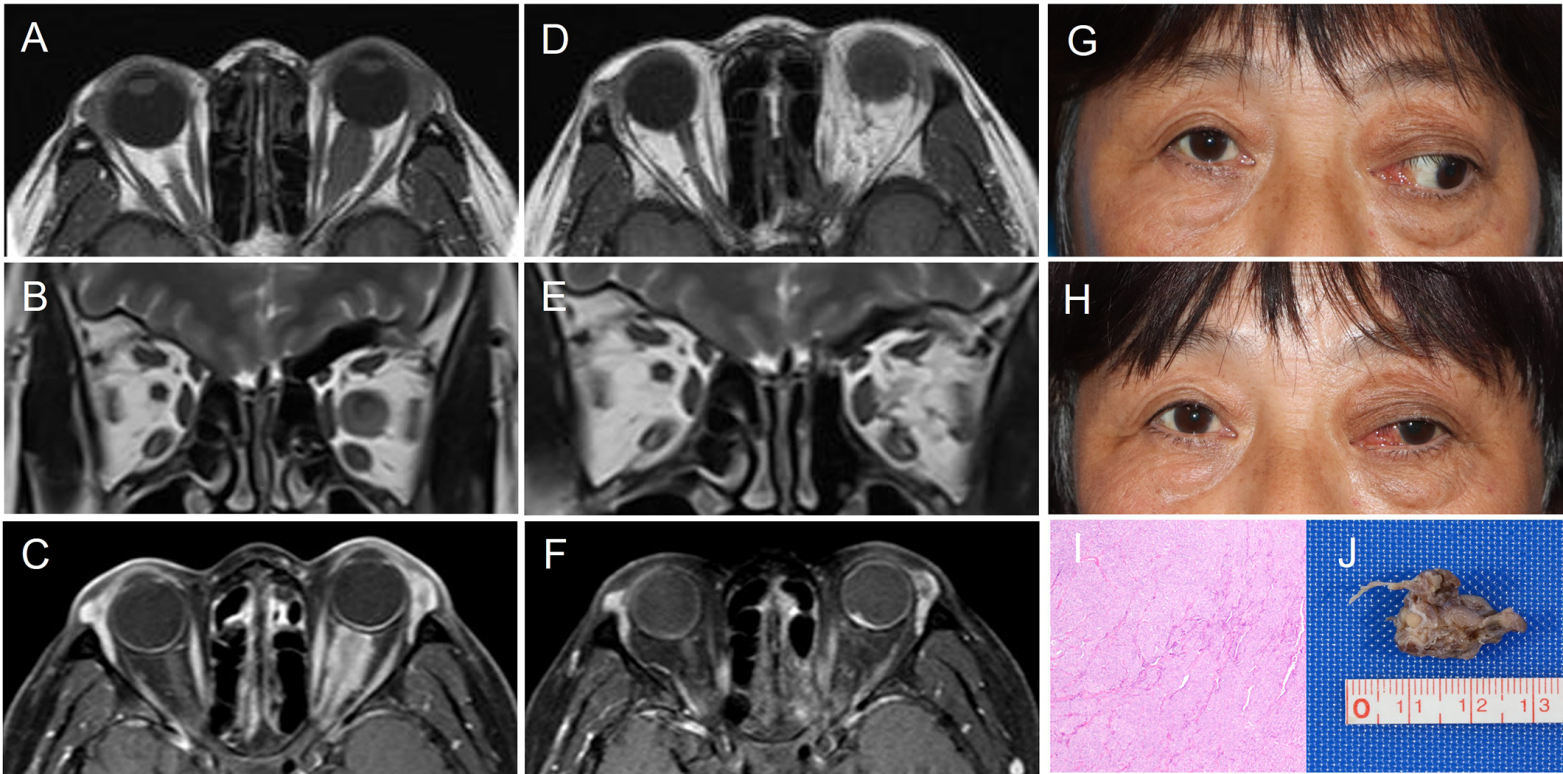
Representative case: a 43-year-old female with longstanding visual loss and proptosis of the left eye. Preoperative MRI, (A)T1-weighted axial MRI, (B)T2-weighted coronal MRI, (C)T1-contrast-enhanced axial MRI. The patient number and corresponding details for this patient (Patient 2) are shown in Table 1. Postoperative MRI demonstrating complete tumor removal, (D)T1-weighted axial MRI, (E)T2-weighted coronal MRI, (F)T1-contrast-enhanced axial MRI. (G, H) Pre- and postoperative appearance at the one month follow up. Resected tumor pathological findings (I) indicated that the tumor was an optic nerve sheath meningioma. The formalin-fixed resected tumor sample (J).
